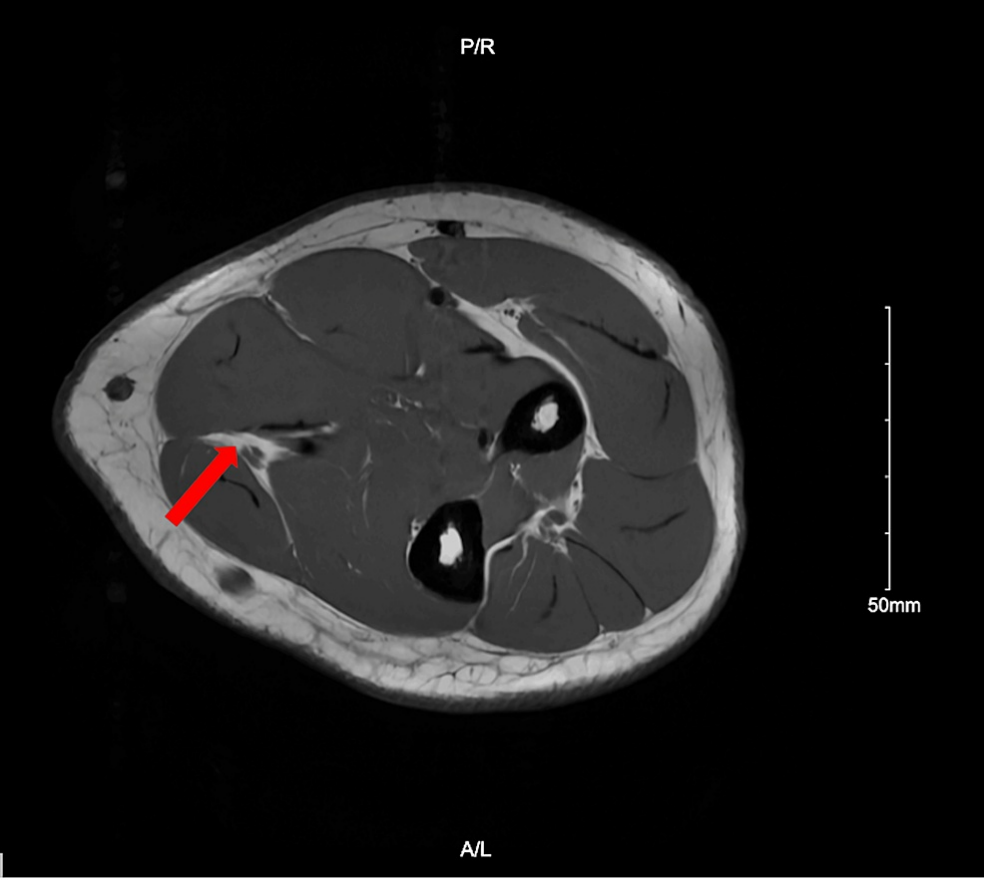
Axial T2 inversion recovery MRI of the left distal 1/3 of the forearm showing nerve sheath tumor, which measures about 5 mm oblique transverse by 8.5 mm proximal-distal by 4 mm. The tumor is within an 8.5 cm segment of the thickened ulnar nerve along the previous resection site.
